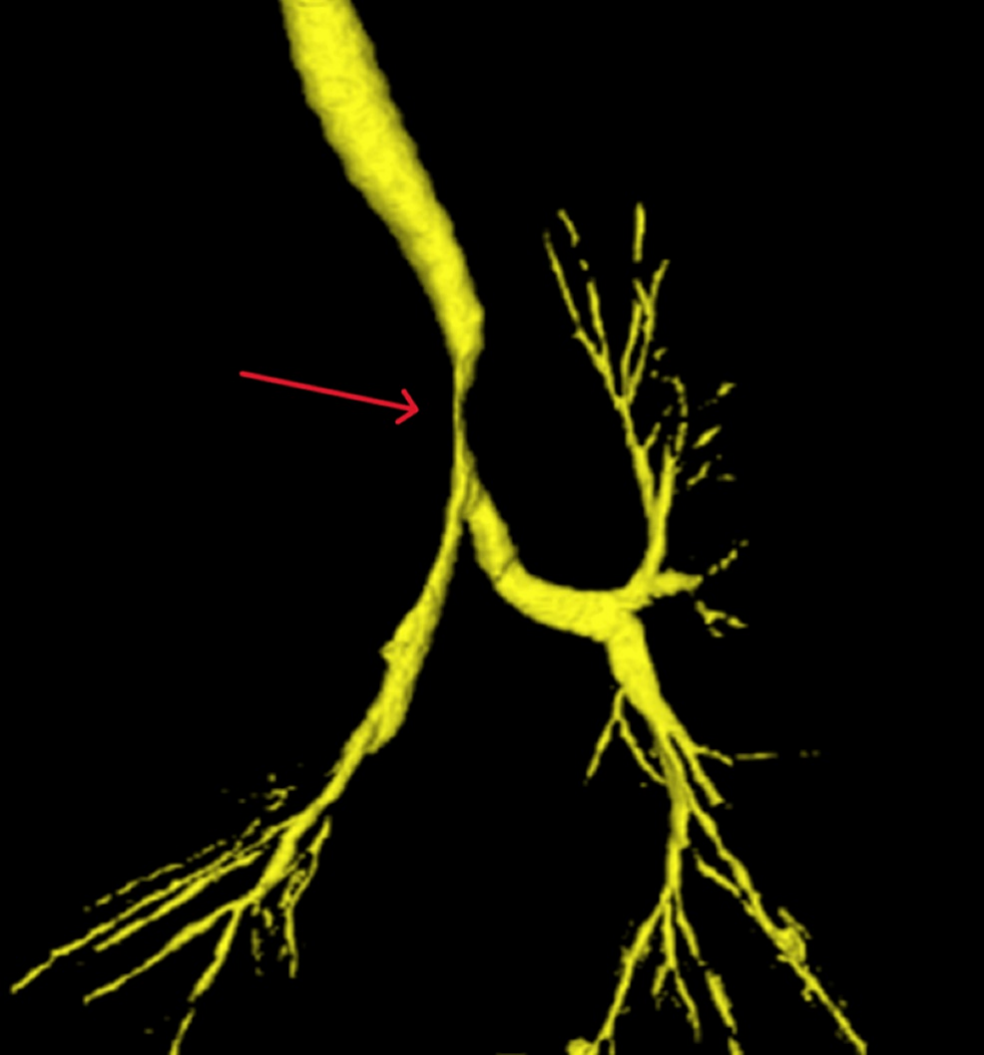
Three-dimensional CT of trachea and bronchi The red arrow indicates the most narrowed part of the trachea. The right lung and trachea are compressed by a large mass.
